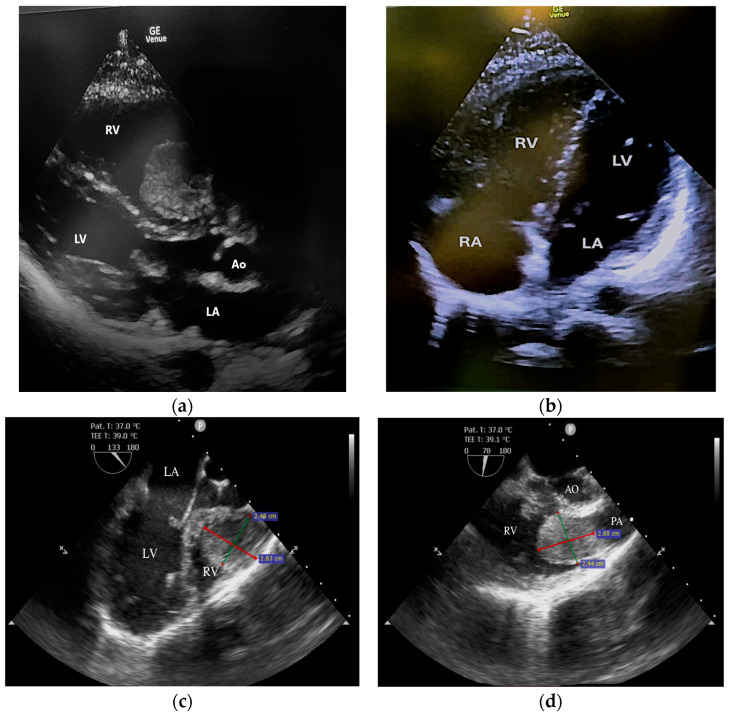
The tumoral mass identified by echocardiography: (a) Transthoracic echocardiography: parasternal long axis view. (b) Transthoracic echocardiography: apical four-chamber view. (c) Transesophageal echocardiography: mid-esophageal short-axis view displaying the tumor in RVOT. (d) Transesophageal echocardiography: mid-esophageal short-axis view displaying the tumor in the main pulmonary artery. RV, right ventricle; RA, right atrium; LV, left ventricle; LA, left atrium; PA, main pulmonary artery; RVOT, right ventricular outflow tract.
